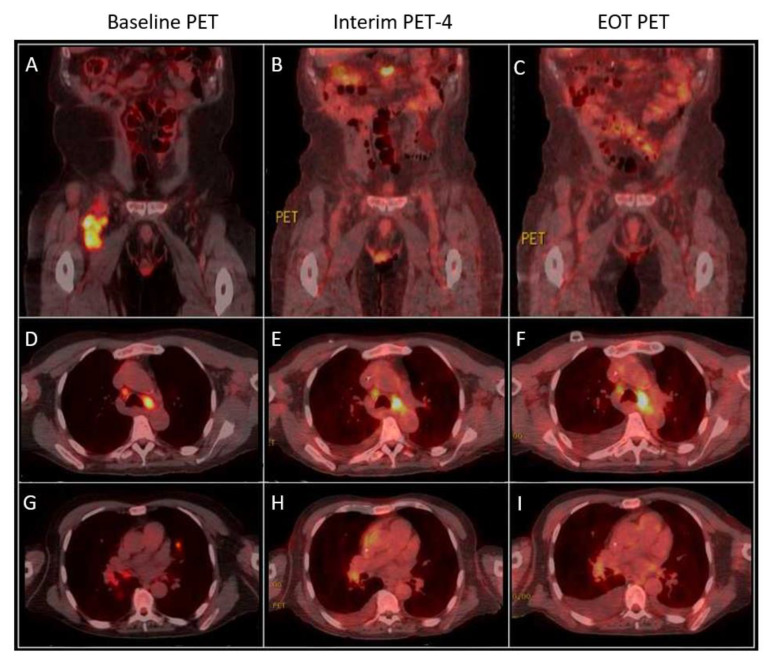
Comparison of baseline, interim, and end-of-treatment (EOT) PET-CT. (A) An abnormal 5.8 × 3.9 cm^2^ soft tissue mass (SUV 11.9) adjacent to the proximal right femur demonstrates near complete resolution after cycle 4 (SUV 1.68) (B) and complete resolution after cycle 6 (C). (D) Enhancing mediastinal adenopathy (max SUV 13.4, Deauville Score 5) with significant improvement (SUV 9.3, Deauville Score 4) after cycle 4 (E) maintained after cycle 6 (Deauville Score 4) (F). (G) Abnormal FDG uptake of numerous subcentimeter bilateral pulmonary nodules (max SUV 8.4) with complete resolution after cycle 4 (H) and maintained after cycle 6 (I).
